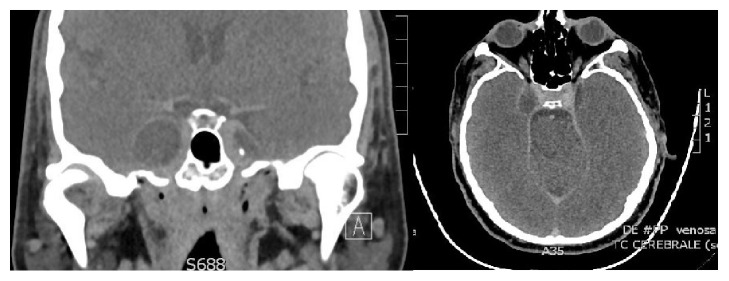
The CT venous phase shows that the cavernous sinus is compressed and medialized by the tumour rather than invaded by it.
